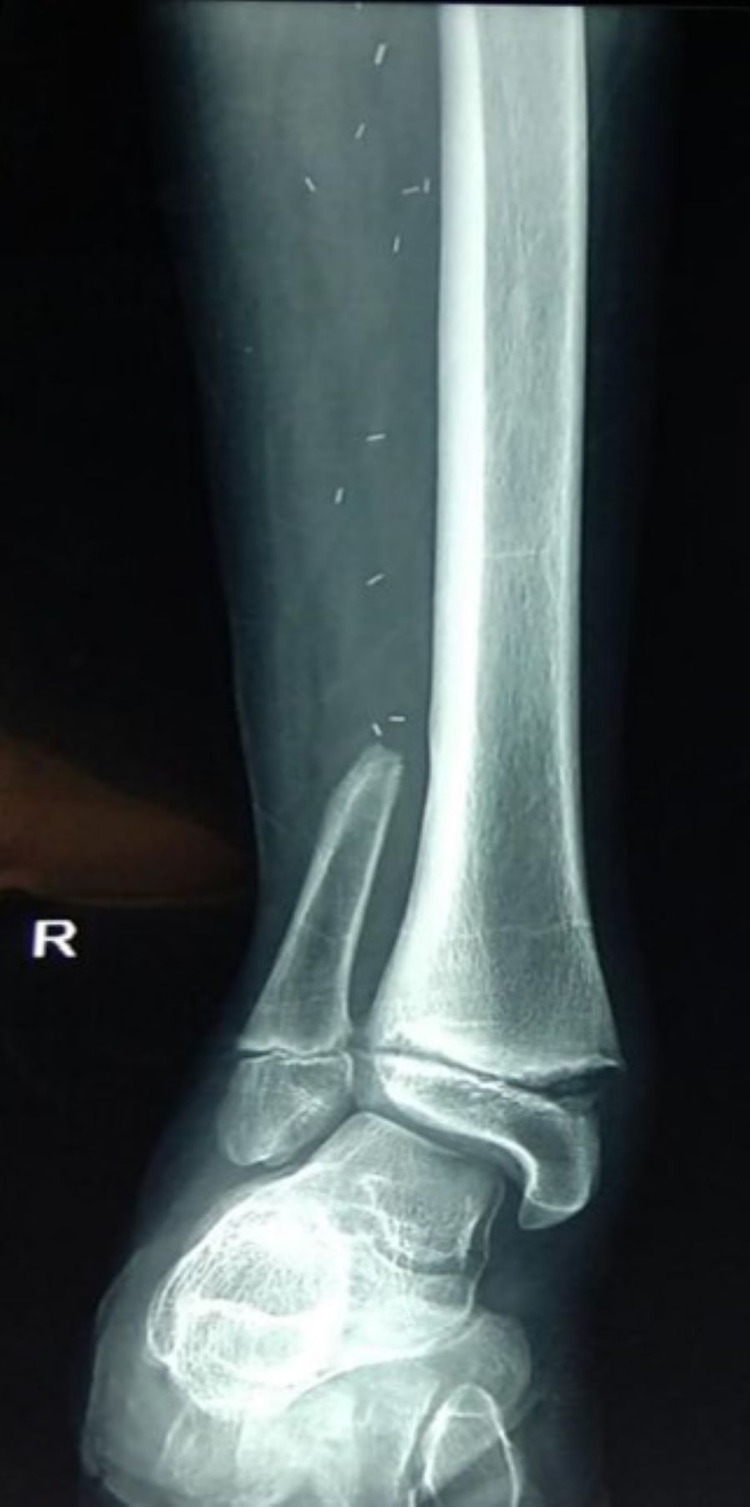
Anteroposterior radiograph of right ankle joint after fibula flap harvest, showing increased subtalar joint angulation.
